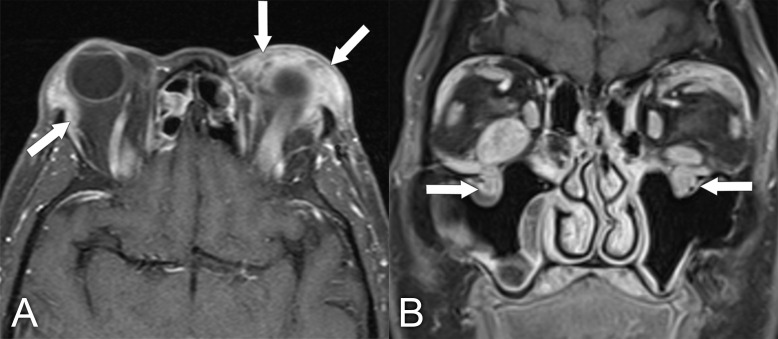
MRI findings in a 61-year-old man who presented with a several-year history of periorbital edema and proptosis. A biopsy of the orbital lesion confirmed a diagnosis of sclerosing orbital inflammatory syndrome, with more than 40% of plasmacytes positive for IgG4 and elevated serum IgG4 levels, making the diagnosis of IgG4-related disease very likely. (A) Axial T1 postcontrast MRI with fat saturation shows diffuse soft tissue inflammation in both orbits (arrows). (B) Coronal T1 postcontrast with fat saturation shows enlargement of the infraorbital nerves bilaterally, which is quite characteristic of IgG4-related disease (arrows).
